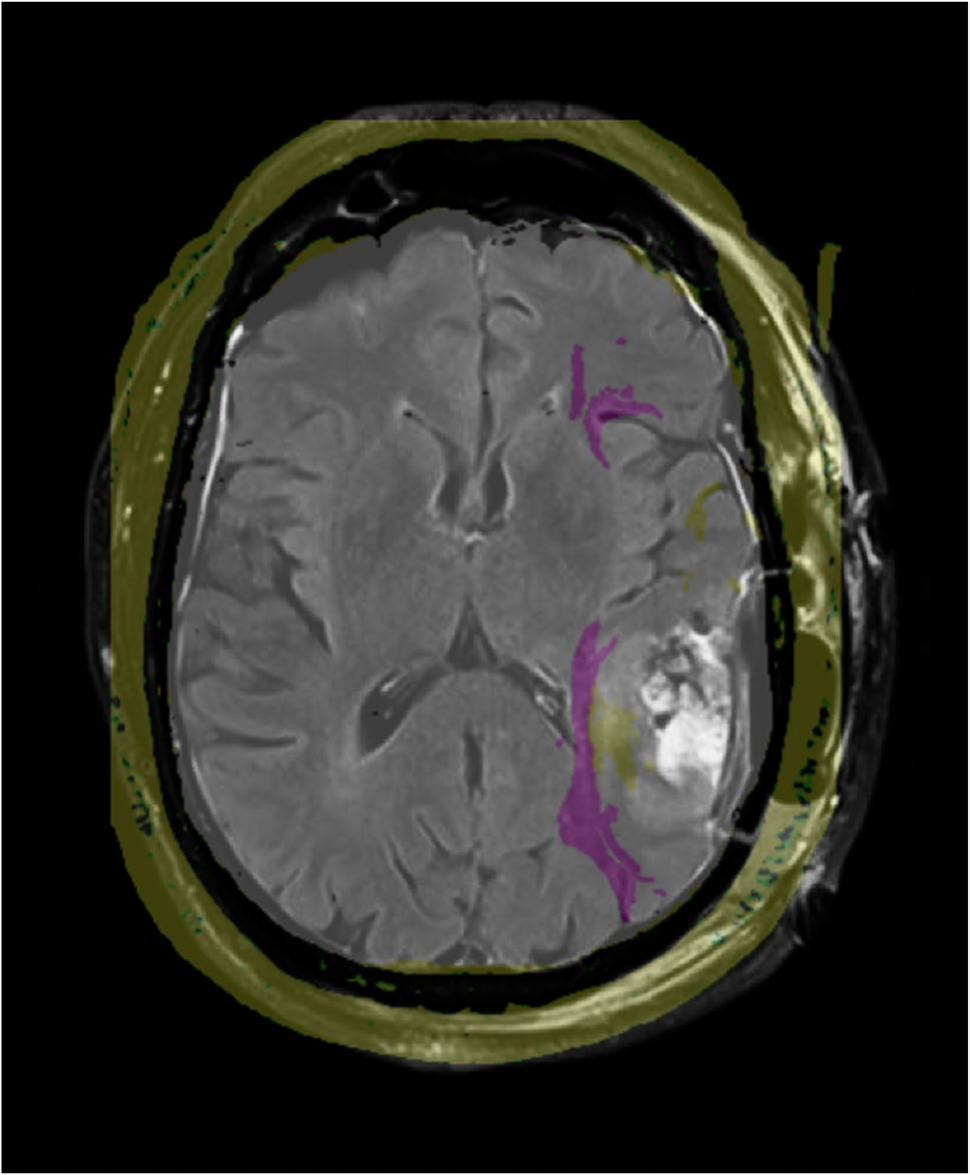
FLAIR sequence of the postoperative magnetic resonance imaging showing gross total resection of the tumor. The preoperative DTI has been superimposed, indicating that the medial resection was stopped at the level of the AF (yellow) and the IFOF (purple)
